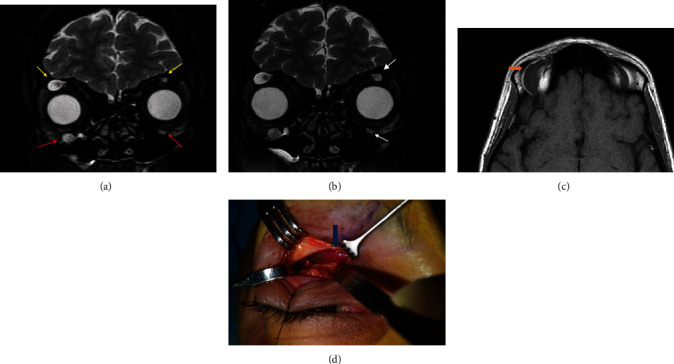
(a) Coronal MRI T2-weighted imaging showed bilateral hyperintense well-defined extraconal masses in the anterosuperior margin of both orbits (yellow arrows) (the greater one on the right side) corresponding to the supraorbital nerves. There was also another extraconal mass in the anteroinferior margin of the right orbit related to the infraorbital nerve, with similar appearance, and smaller on the left side (red arrows). (b) Growth of lesions in the left orbit (white arrows). (c) MRI axial T1-weighted imaging demonstrated the right anterosuperior tumor with isointensity relative to muscle with a fusiform morphology (orange arrow). (d) Intraoperative image of the lesion (blue arrow).
